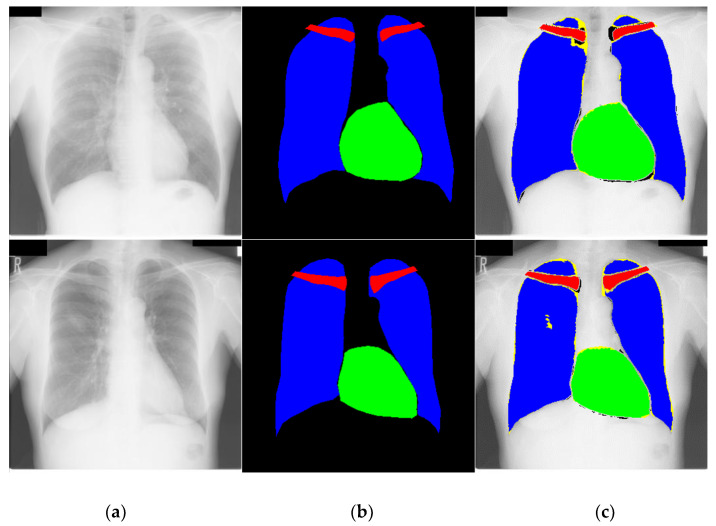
Examples of chest-related organs by CardioNet: (a) original chest PA X-ray image; (b) image with a ground-truth mask; (c) CardioNet predicted mask.
